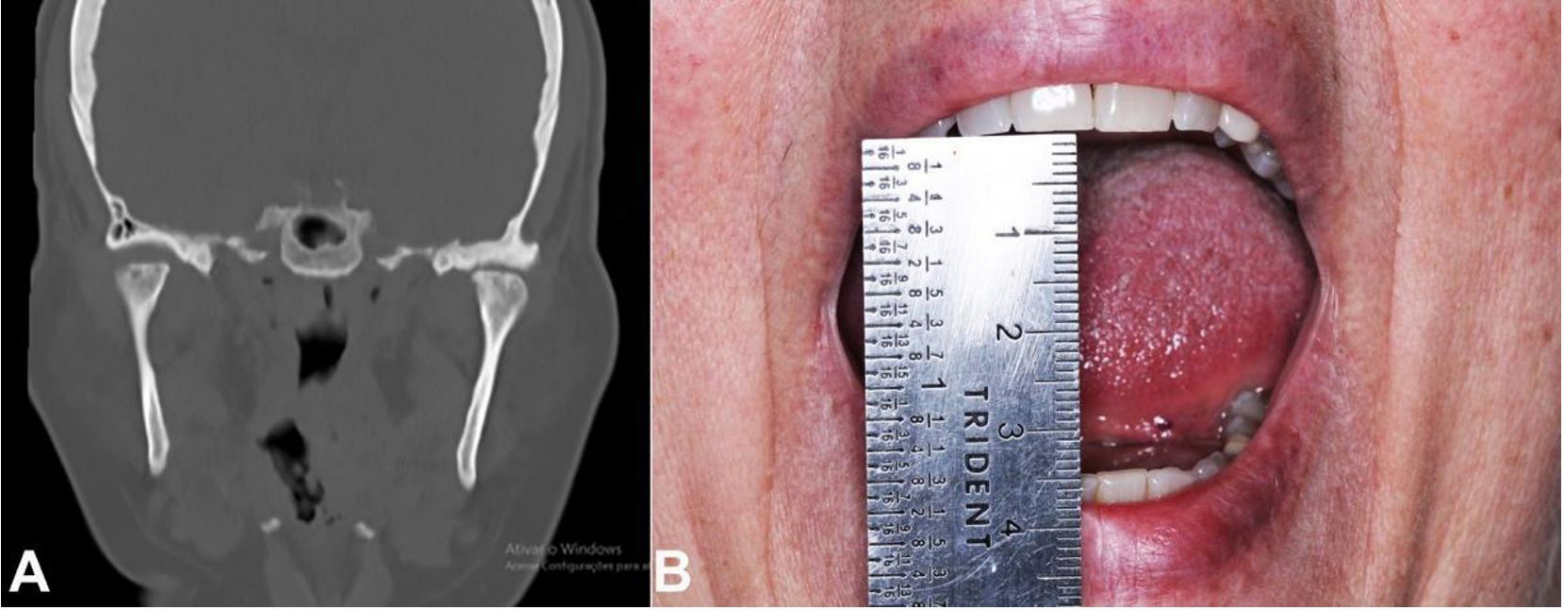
A – 13-months post-operative CT image showing the absence of loose bodies; B – Mouth opening improvement after 13-months post-operatory.
